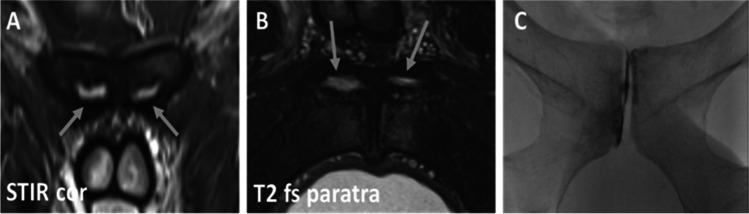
Example of bilateral atypical cleft signs of one patient in MRI (arrows in A and B) and lack of corresponding pattern in symphysography (C). The atypical cleft signs might represent PLAC injuries
